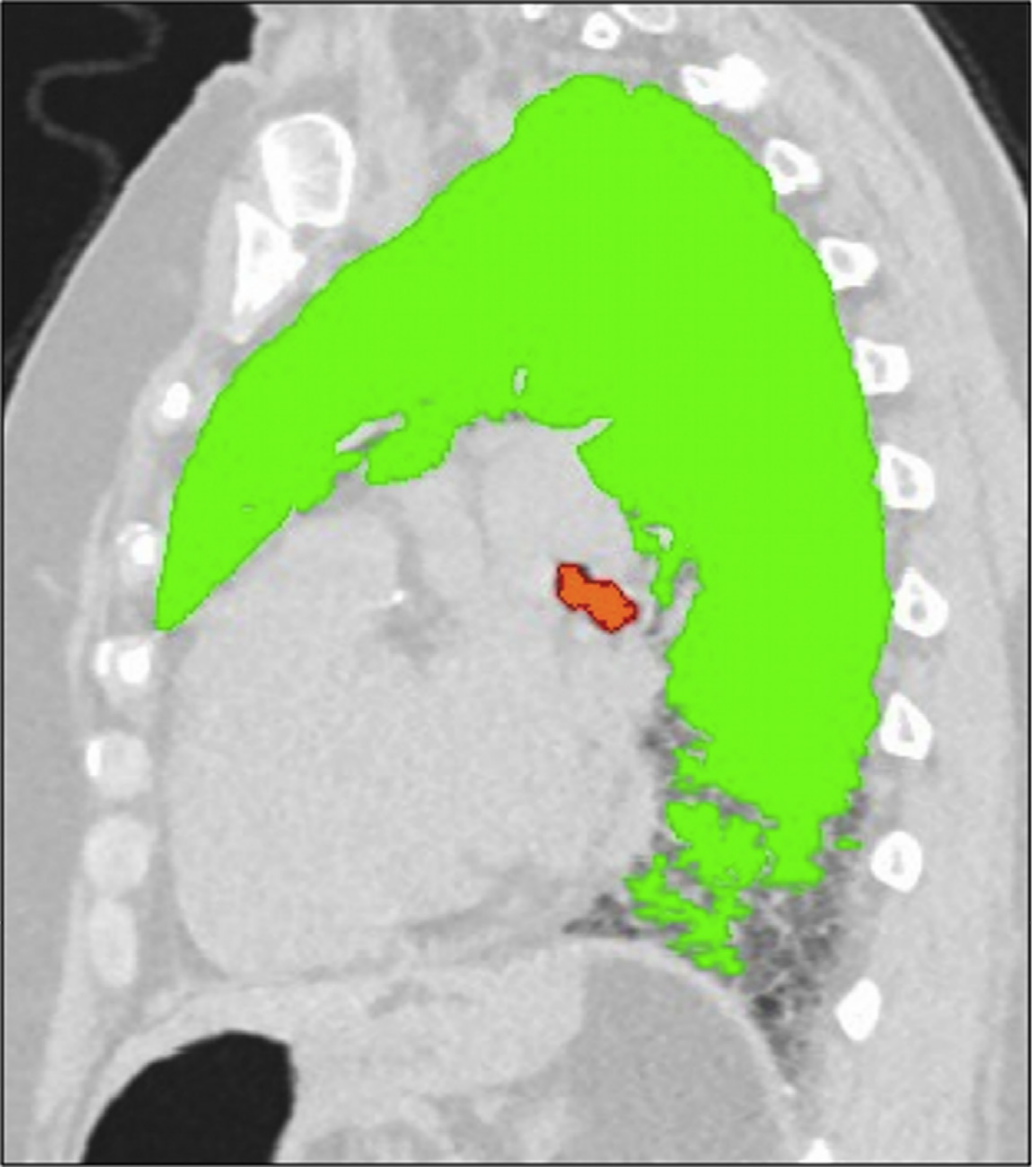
Visualization of the fully automatic lung parenchyma segmentation as obtained by in-house YACTA software. Sagittal reconstruction image of a non-enhanced MDCT scan obtained from a patient suffering from idiopathic pulmonary fibrosis (IPF) not included in the current trial. YACTA software automatically segmented lung parenchyma and trachea-bronchial tree, emphasized as green and orange overlay respectively (window width: 1600 HU; level: -600 HU). Note that the segmentation algorithm fails to segment portions of the lung parenchyma in the sub-pleural space of the recessus, due to its similar density to the chest wall. (MDCT = multidetector computed tomography).
